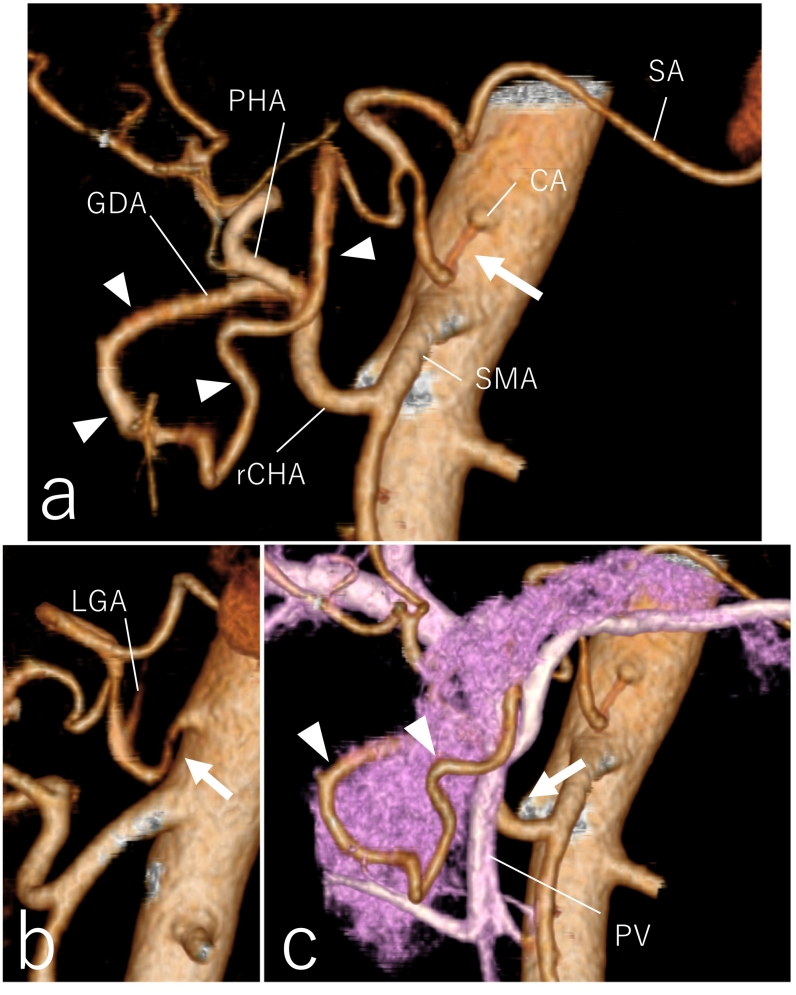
Preoperative CT angiography findings. (a) Front view. Stenosis of the origin of the CA is observed (arrow). The rCHA arises from the SMA and the GDA branches from the rCHA. The collateral pathway of the pancreatic head is developed (arrowhead). (b) Lateral view. Focal stenosis in the proximal celiac axis is described as having a “hooked appearance” (arrow). (c) Three-dimensional CT shows the rCHA passing behind the PV (allow), and the collateral artery runs around the head of the pancreas (arrowhead). CT: computed tomography; CA: celiac artery, rCHA: replaced common hepatic artery, SMA: superior mesenteric artery, GDA: gastroduodenal artery, SA: splenic artery, PHA: proper hepatic artery, LGA: left gastric artery, PV: portal vein.
